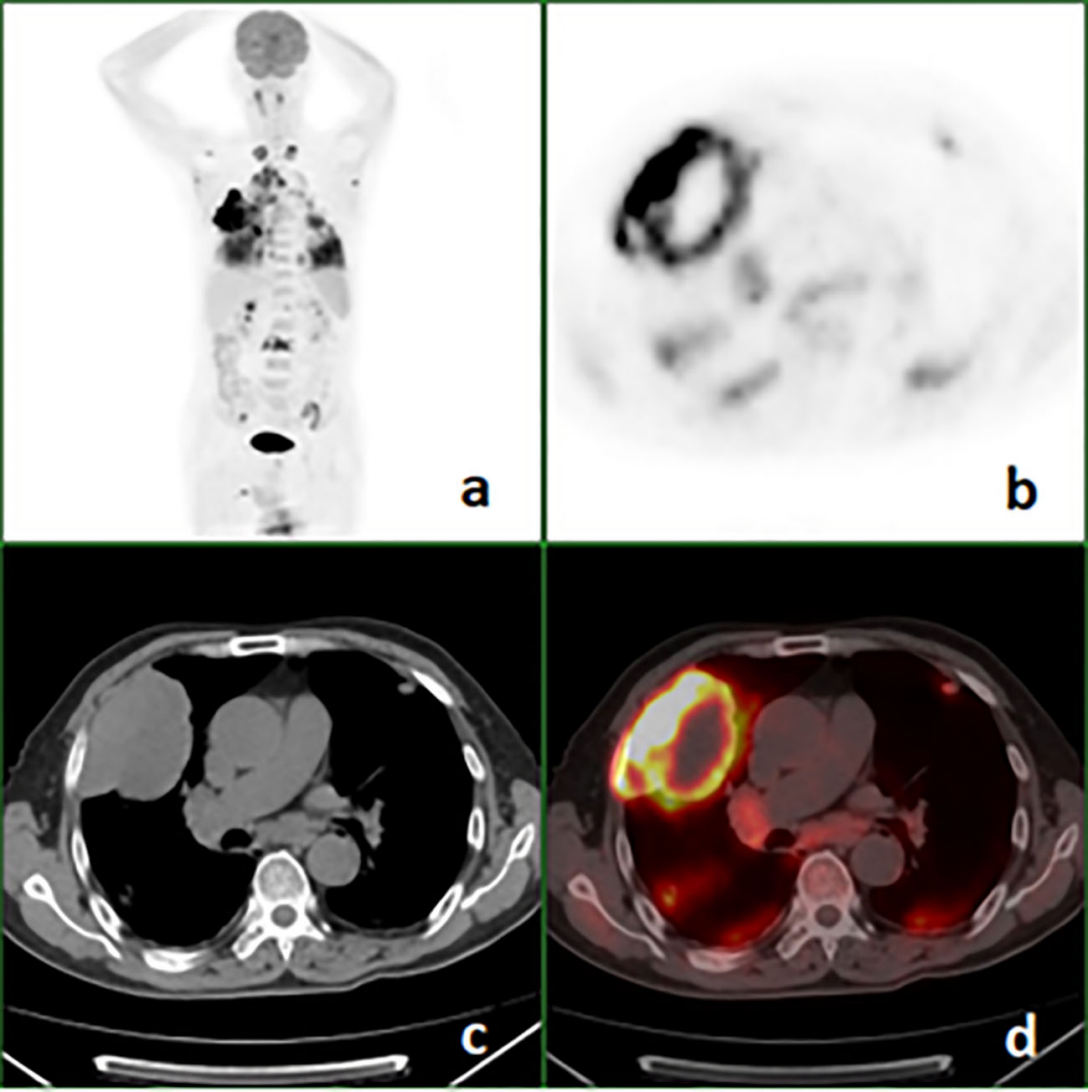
^18^F-FDG PET/CT images of a 68-year-old man with pulmonary sarcomatoid carcinoma showing a large mass in the right upper lung with broad pleural invasion, Lung and bone metastases. (A) PET maximal intensity projection (MIP); (B), PET; (C), axial CT (mediastinal window); (D), fusion image.
